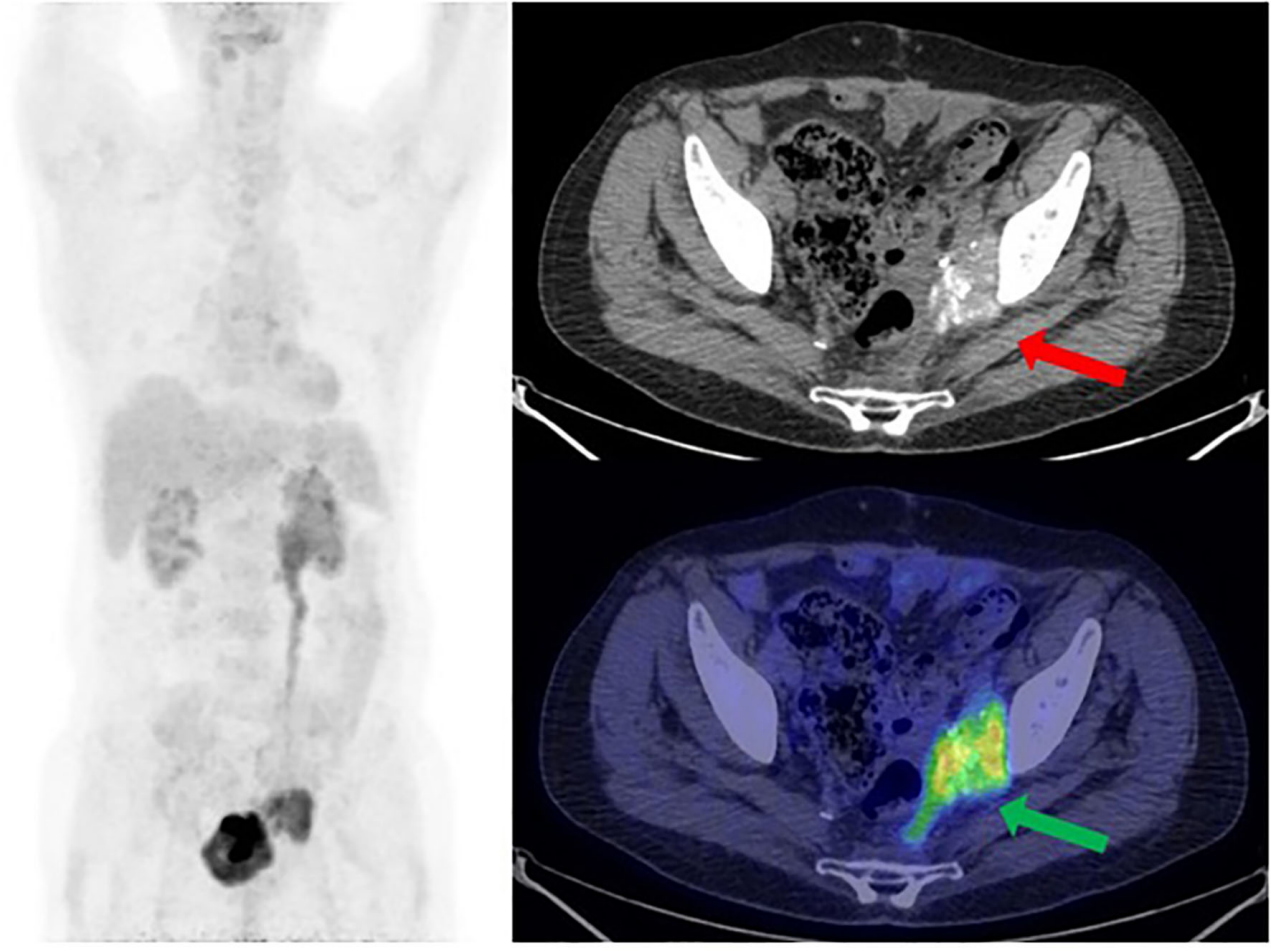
[18F] FDG PET/CT MIP reconstruction and transaxial image (performed at the University Hospital of Bari) showed the recurrence of disease at the left side of the pelvis, near the bladder, with a size of 3.7 × 4.8 cm and an mSUV of 7.7 (green arrow). Note the psammomatous bodies (concentric calcifications, a consequence of apoptotic cell desquamation—red arrow), a typical feature of ovarian tumors, which confirm the Mullerian origin of this man’s tumor.
